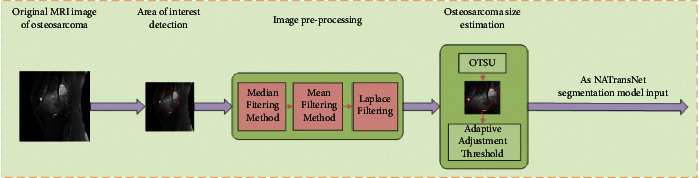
The input osteosarcoma MRI image is segmented into the suspected area of osteosarcoma to remove the irrelevant background in the osteosarcoma and reduce the network burden.
